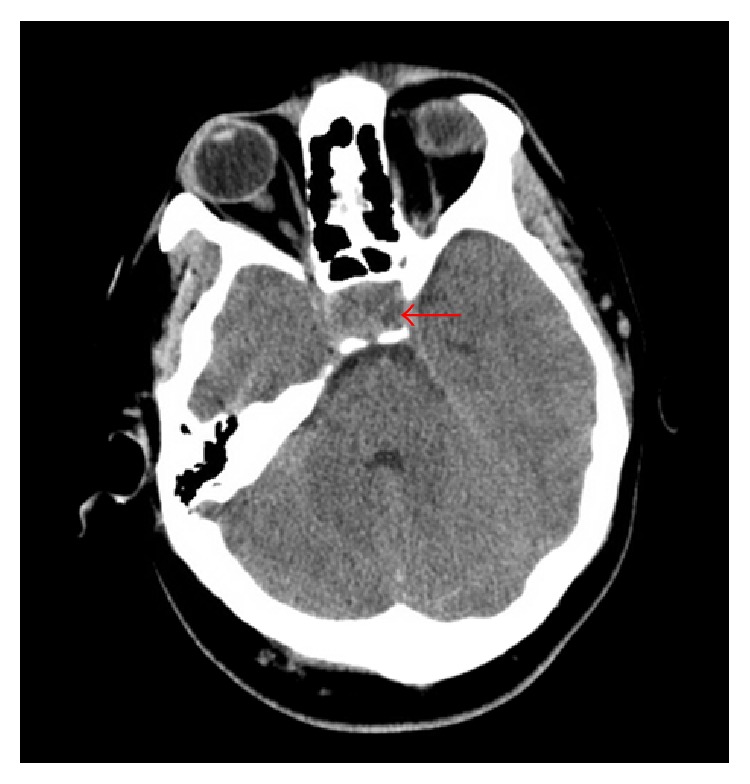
Noncontrast head CT. Red arrow indicating intra-/parasellar mass.
